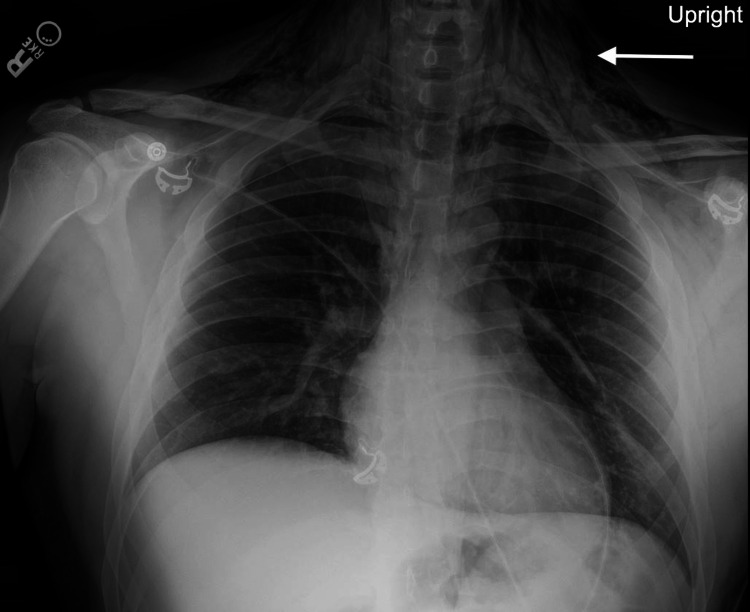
Initial chest radiograph with an arrow pointing to subcutaneous air in the neck
